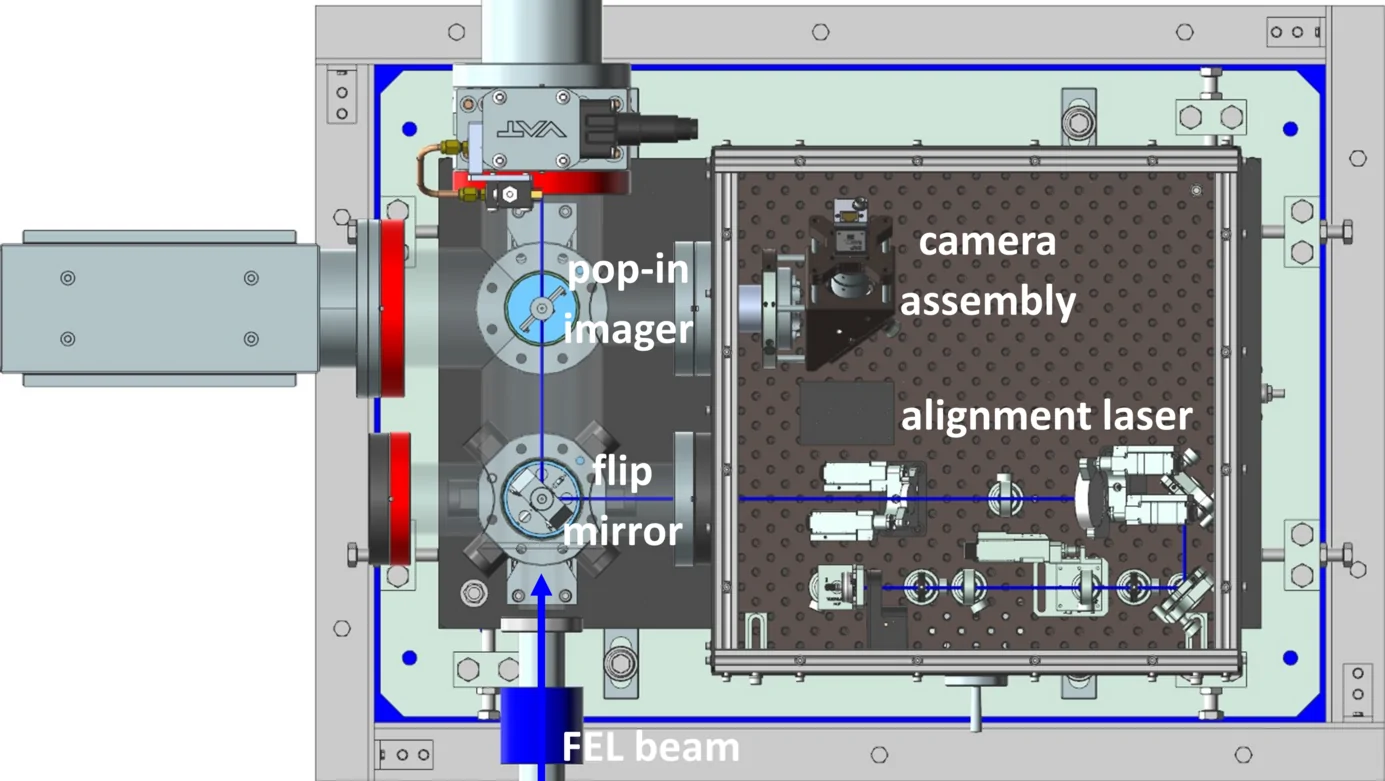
Top view of the mechanical layout of the alignment laser system (ALAS) positioned upstream of the KB X-ray optics chamber. The XFEL beam enters from below (CF 40 flange) and traverses two four-way-cross sections with motorized manipulators: A flip mirror injects an optical alignment laser parallel to the XFEL path, while pop-in fluorescent screens can be inserted to monitor both beams.
